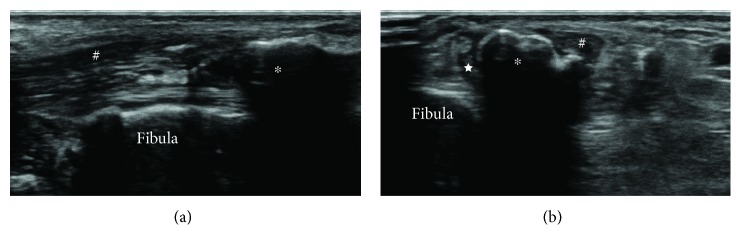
Ultrasonographic images showed an elliptical mass with an echoic shadow on the affected side of the peroneal tendon sheath. (a) Longitudinal image and (b) transverse image. ^∗^Heterotopic ossification. ^#^Peroneus longus tendon. ^★^Peroneus brevis tendon.
